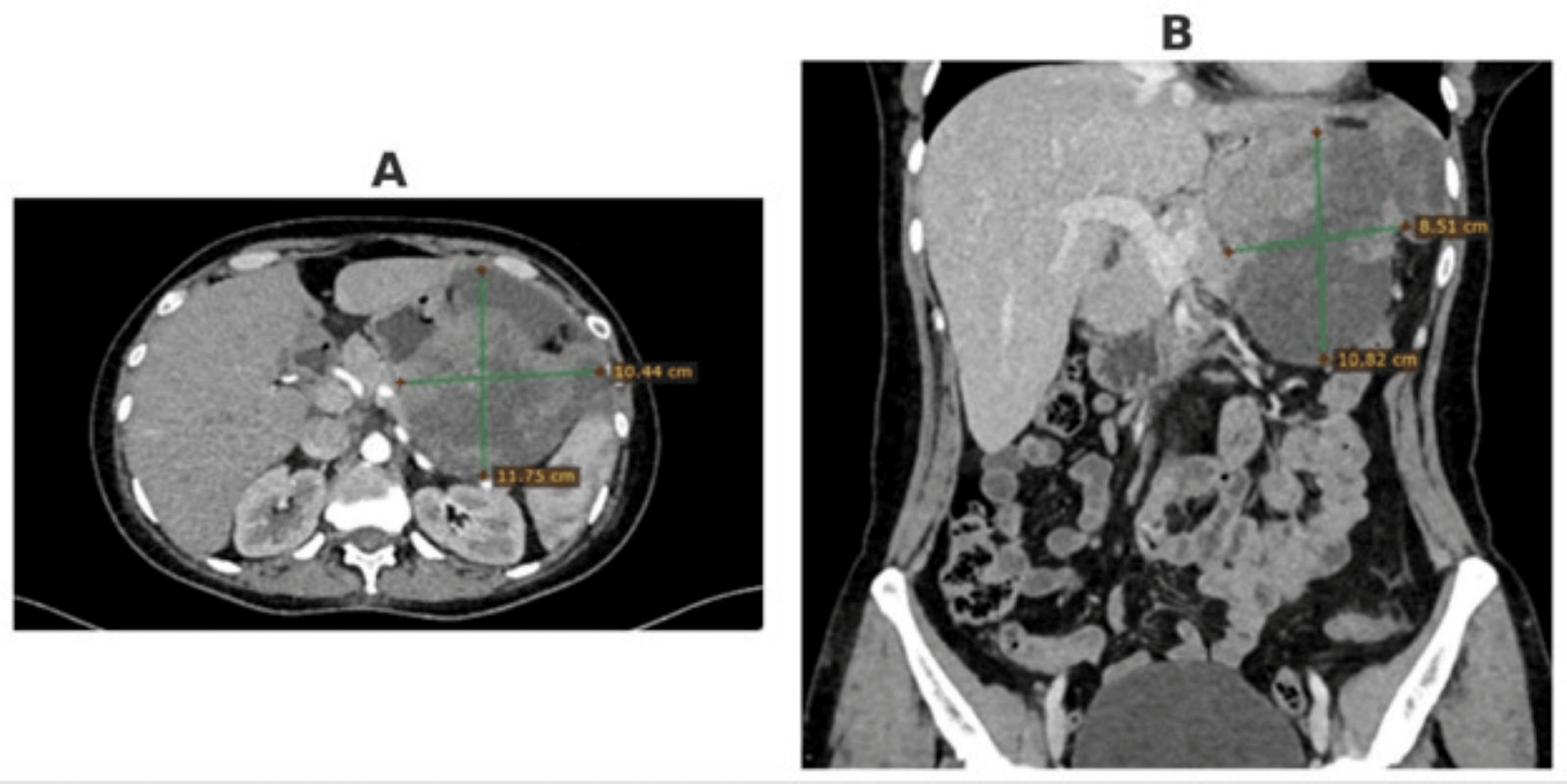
Computed tomography of the abdomen with intravenous contrast. (A) Axial view, (B) Coronal view.
